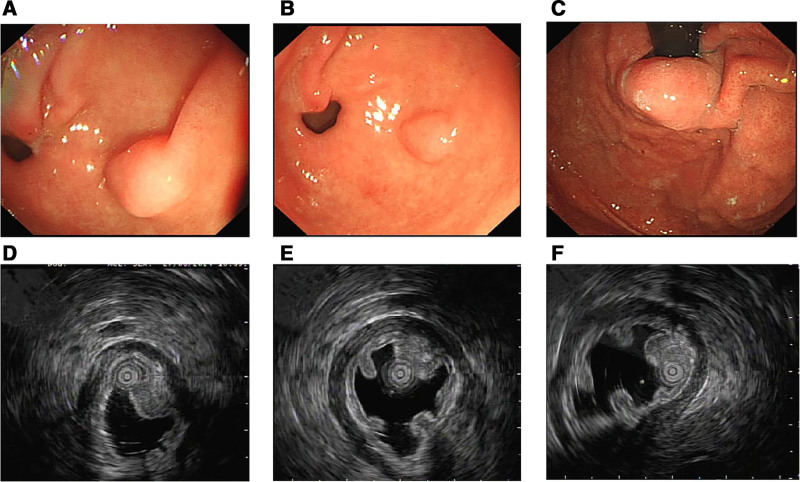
(A and B) show a submucosal protrusion in the gastric antrum under white light endoscopy, with a smooth mucosal surface and a slight depression in the center. (D–F) display the gastric antral protrusion under endoscopic ultrasound (EUS), which is located in the muscularis mucosa and submucosa, presenting as a slightly hyperechoic lesion with a size of approximately 10.7 mm × 7.7 mm. The lesion has relatively clear borders, while the demarcation from the muscularis propria is unclear in some layers. The remaining mucosal and outer layers are smooth. (F) reveals that the lesion contains duct-like structures. (C) shows a submucosal tumor at the cardiac orifice.
